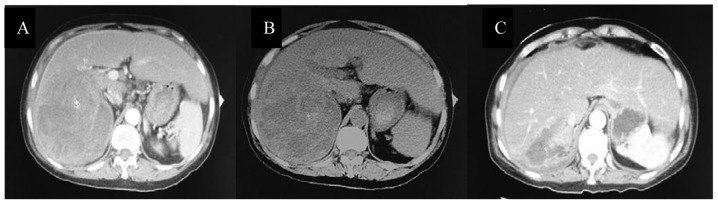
(A) Contrast-enhanced CT of the abdomen showing an enhancing mass (arrow) in the right half of the liver prior to surgery. (B) Delayed-phase CT of the abdomen showing a mass (arrow) of homogeneous lower attenuation in the right half of the liver prior to surgery. (C) Contrast-enhanced CT of the abdomen showing that the mass was no longer present in the right hemi-liver post-operatively. CT, computed tomography.
